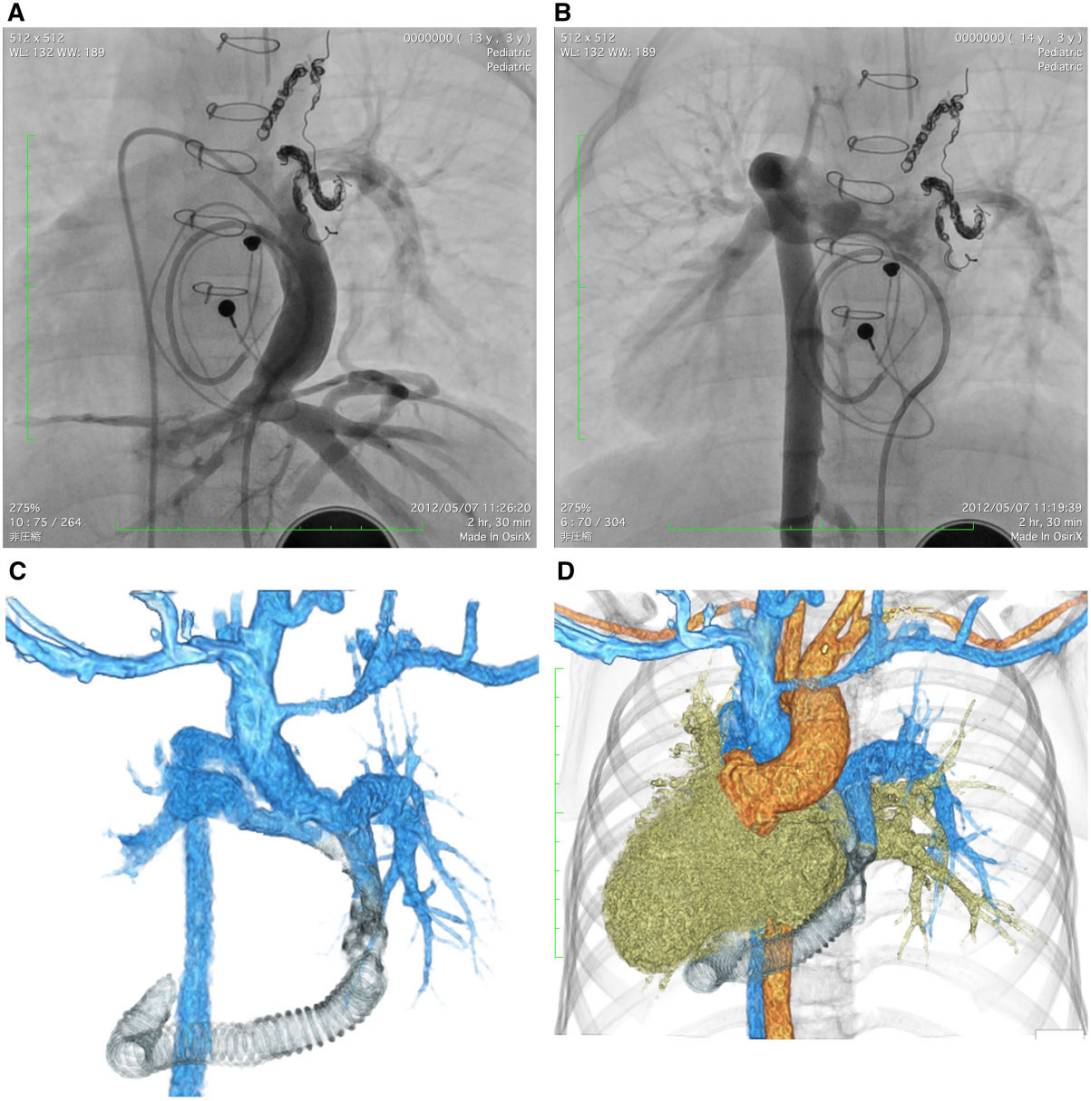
(A and B) Angiography findings after the hepatic vein-to-pulmonary artery conduit procedure. (C and D) Computed tomography findings after the hepatic-to-azygos vein redirection.
